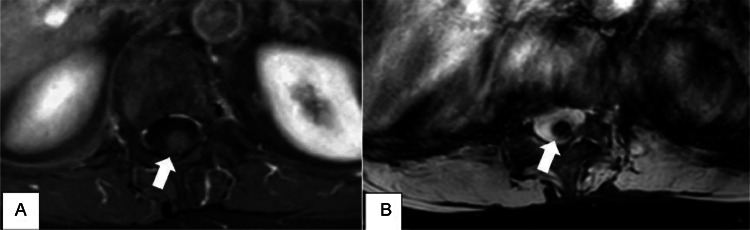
(A) Axial T1-weighted-SPIR MR image with intravenous contrast material showing slight gadolinium enhancement of the lesion (arrow). (B) Axial T2-weighted-FFE MRI image showing a perilesional signal drop due to magnetic susceptibility artefacts, more pronounced in GRE sequences (arrow).
